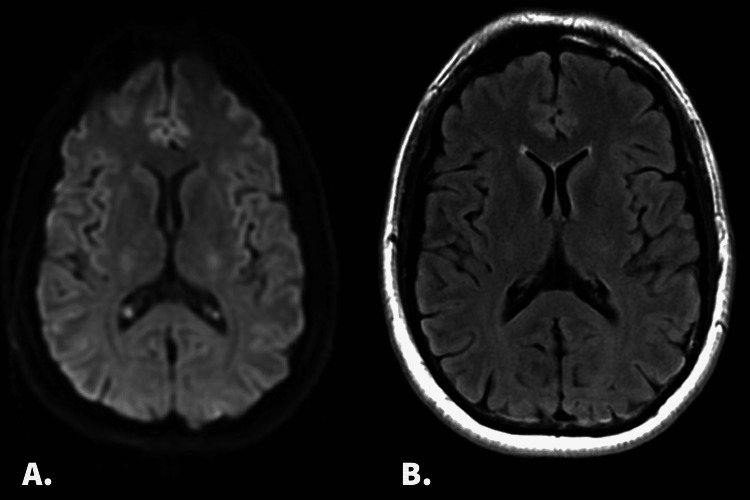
Patient’s MRI imaging sequences The diffusion-weighted imaging (DWI) sequence in Panel A is without diffusion restriction that would suggest an acute or subacute ischemic infarct. The T2 sequence in Panel B is without significant changes that would suggest prior ischemic infarcts or underlying chronic microvascular white matter disease.
